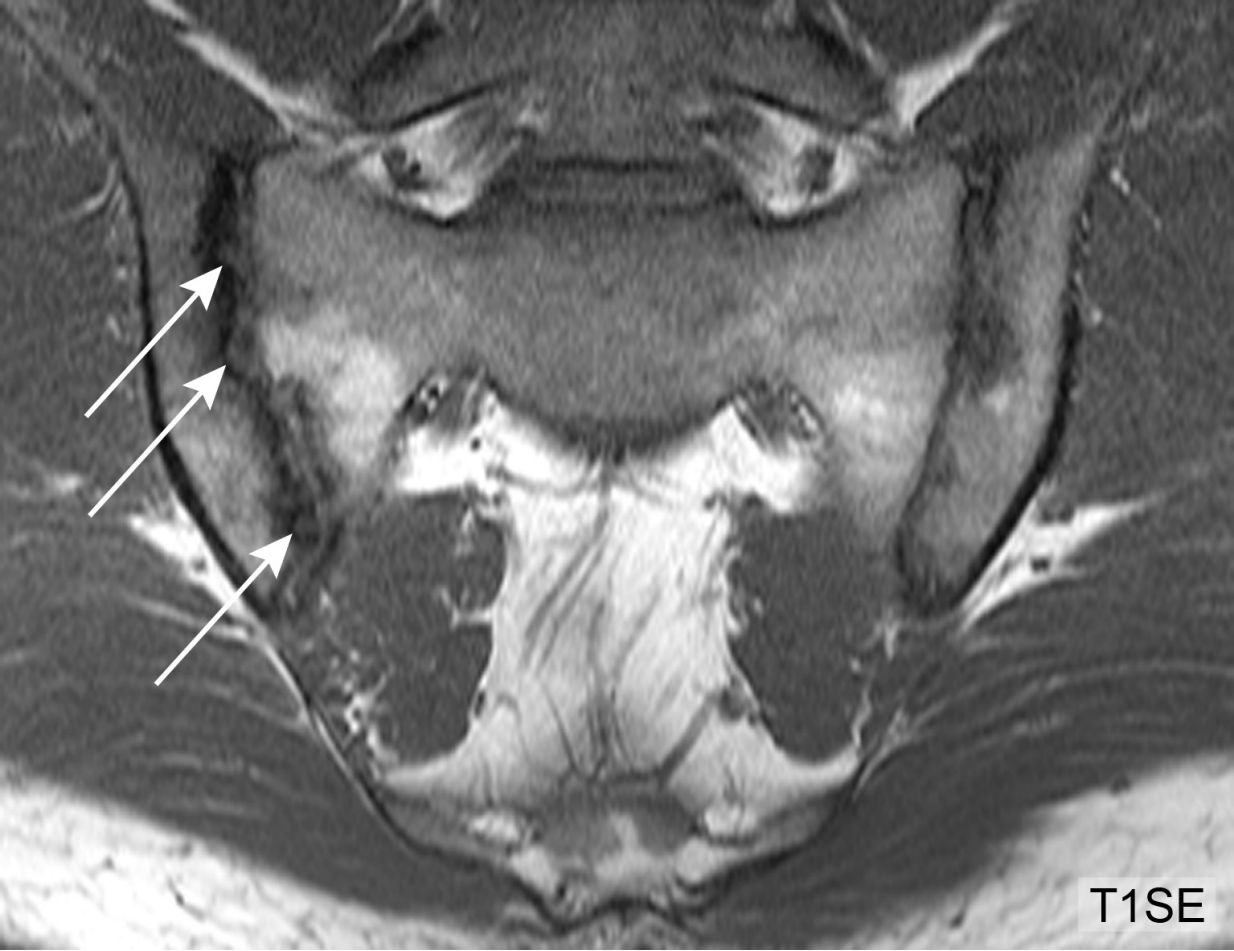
Extended erosion (T1SE sequence). HLA B27 positive, 28-year-old male AS patient with inflammatory back pain for five years. The right ilium shows erosion which extends across the entire joint surface (arrows). HLA-B27, human leucocyte antigen B27; T1SE, T1-weighted spin echo sequence.
